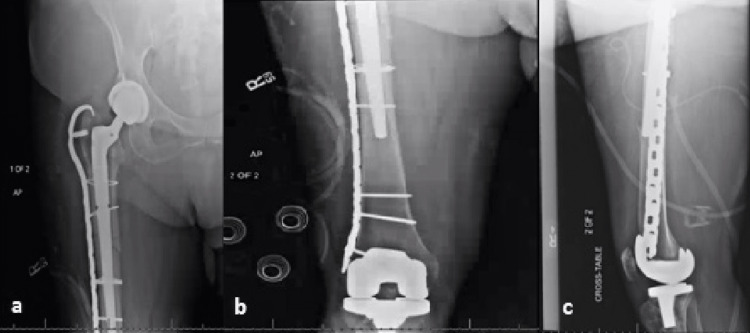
Postoperative radiographs including right proximal femur AP (a), distal femur AP (b), and lateral (c) views. Radiographs depict fracture fixation with a diaphyseal fitting tapered femoral stem, cerclage wires, and lateral trochanteric hook plate. Anterior cortical perforation can be visualized on the lateral radiograph.
